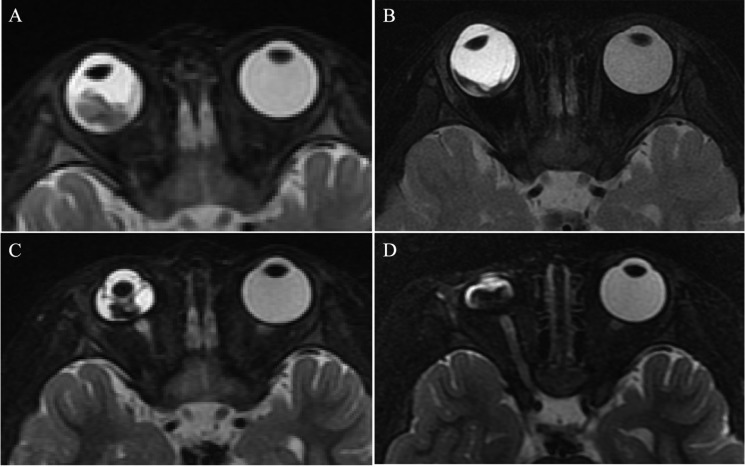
Retinoblastoma diagnosed in a 16-month-old female (patient 4) (A–D) The axial T2-weighted MRI. The right eyeball with retinoblastoma had a size equal to the contralateral eyeball before treatment (A), and became larger in size than the contralateral eyeball in the second month after three cycles of IAC (B). Eyeball atrophy was found in the fifth month after three cycles of IAC (C) and worsened in the tenth month (D).
